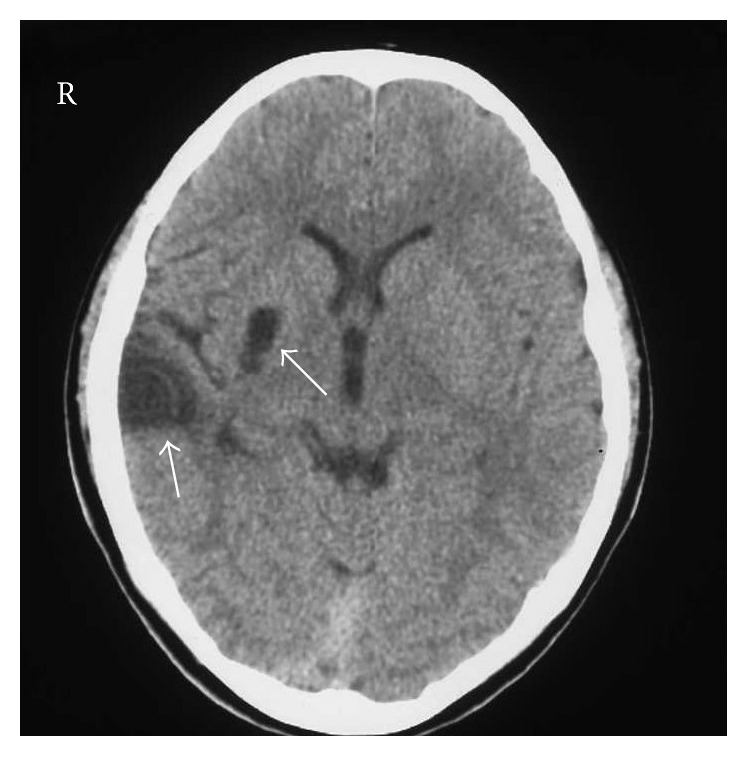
Brain lesion in computed tomography (CT). Low-density areas (arrows) in the right putamen and temporal lobe are observed.
